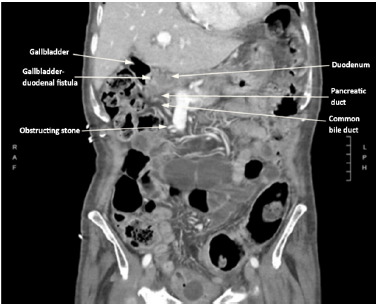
Computed tomography coronal view of a gas-containing gallbladder with fistulous connection to the proximal duodenum. There are common bile duct and main pancreatic duct dilatation due to an obstructing stone within the distal common bile duct.
